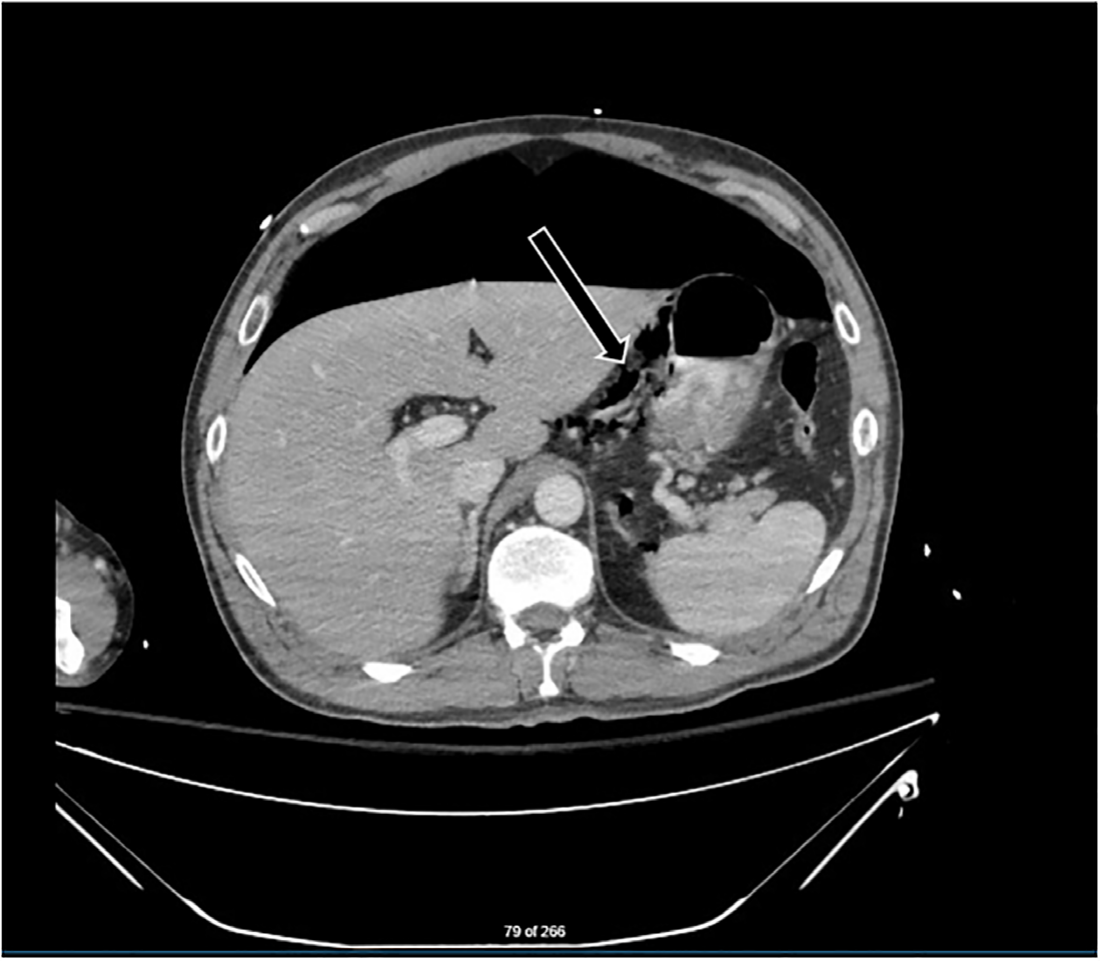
Computed tomography of the abdomen and pelvis with oral contrast at the level of the stomach performed after needle decompression. Large pneumoperitoneum was still present but without tension. Arrow points to air bubbles seen along lesser gastric curvature suggesting location of perforation.
